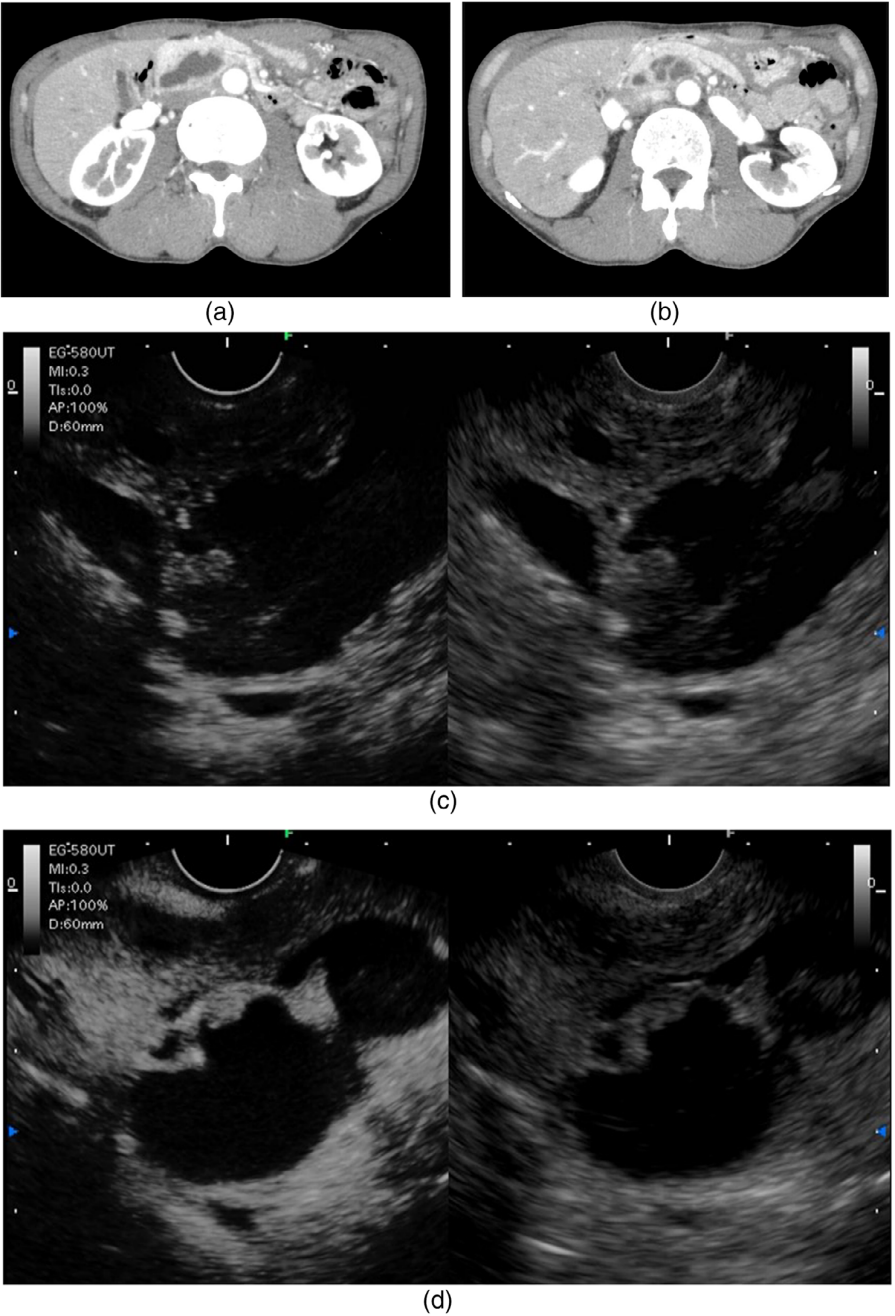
Contrast‐enhanced harmonic endoscopic ultrasonography (CH‐EUS) and contrast‐enhanced computed tomography (CE‐CT) for the diagnosis of mural nodules in intraductal papillary mucinous neoplasm (IPMN). (a) CT findings of mixed IPMN of the pancreatic head. The dilated main pancreatic duct and multilocular cystic lesions adjacent to the main pancreatic duct are seen. (b) CT findings of mixed IPMN of the pancreatic head. Septal structures are observed within multilocular cystic lesions. (c) CH‐EUS (before injection of Sonazoid). Right: reference B‐mode image, Left: contrast‐enhanced harmonic mode. The B‐mode and pre‐contrast harmonic EUS images show a hyperechoic polypoid lesion within the cyst. (d) CH‐EUS (after injection). Right: Reference B‐mode image; Left: Harmonic mode with contrast In CH‐EUS, the contrast effect is seen in the septal wall within the cyst and in the mural lesion, allowing the precise diagnosis of a mural nodule.
